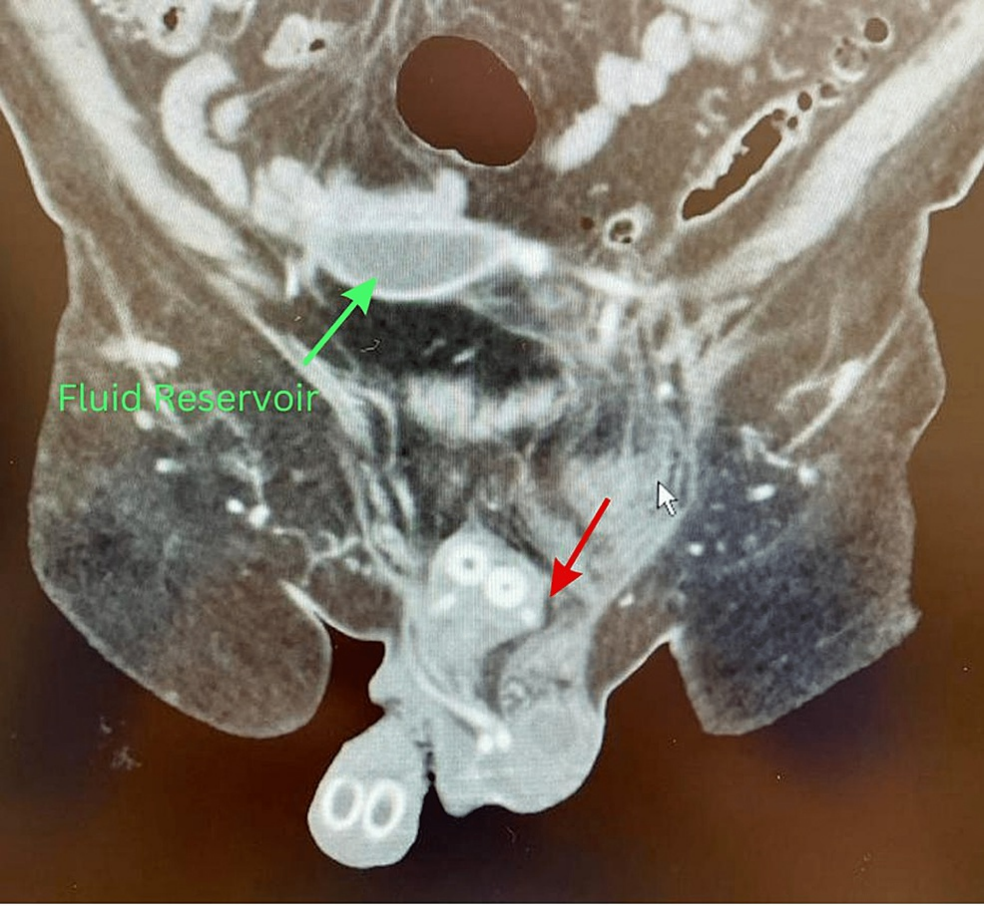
CT scan of the pelvis and lower abdomen. Sagittal plane of the CT scan. The red arrow indicates the left inguinal hernia, and the green arrow indicates the fluid reservoir in the abdominal cavity.
